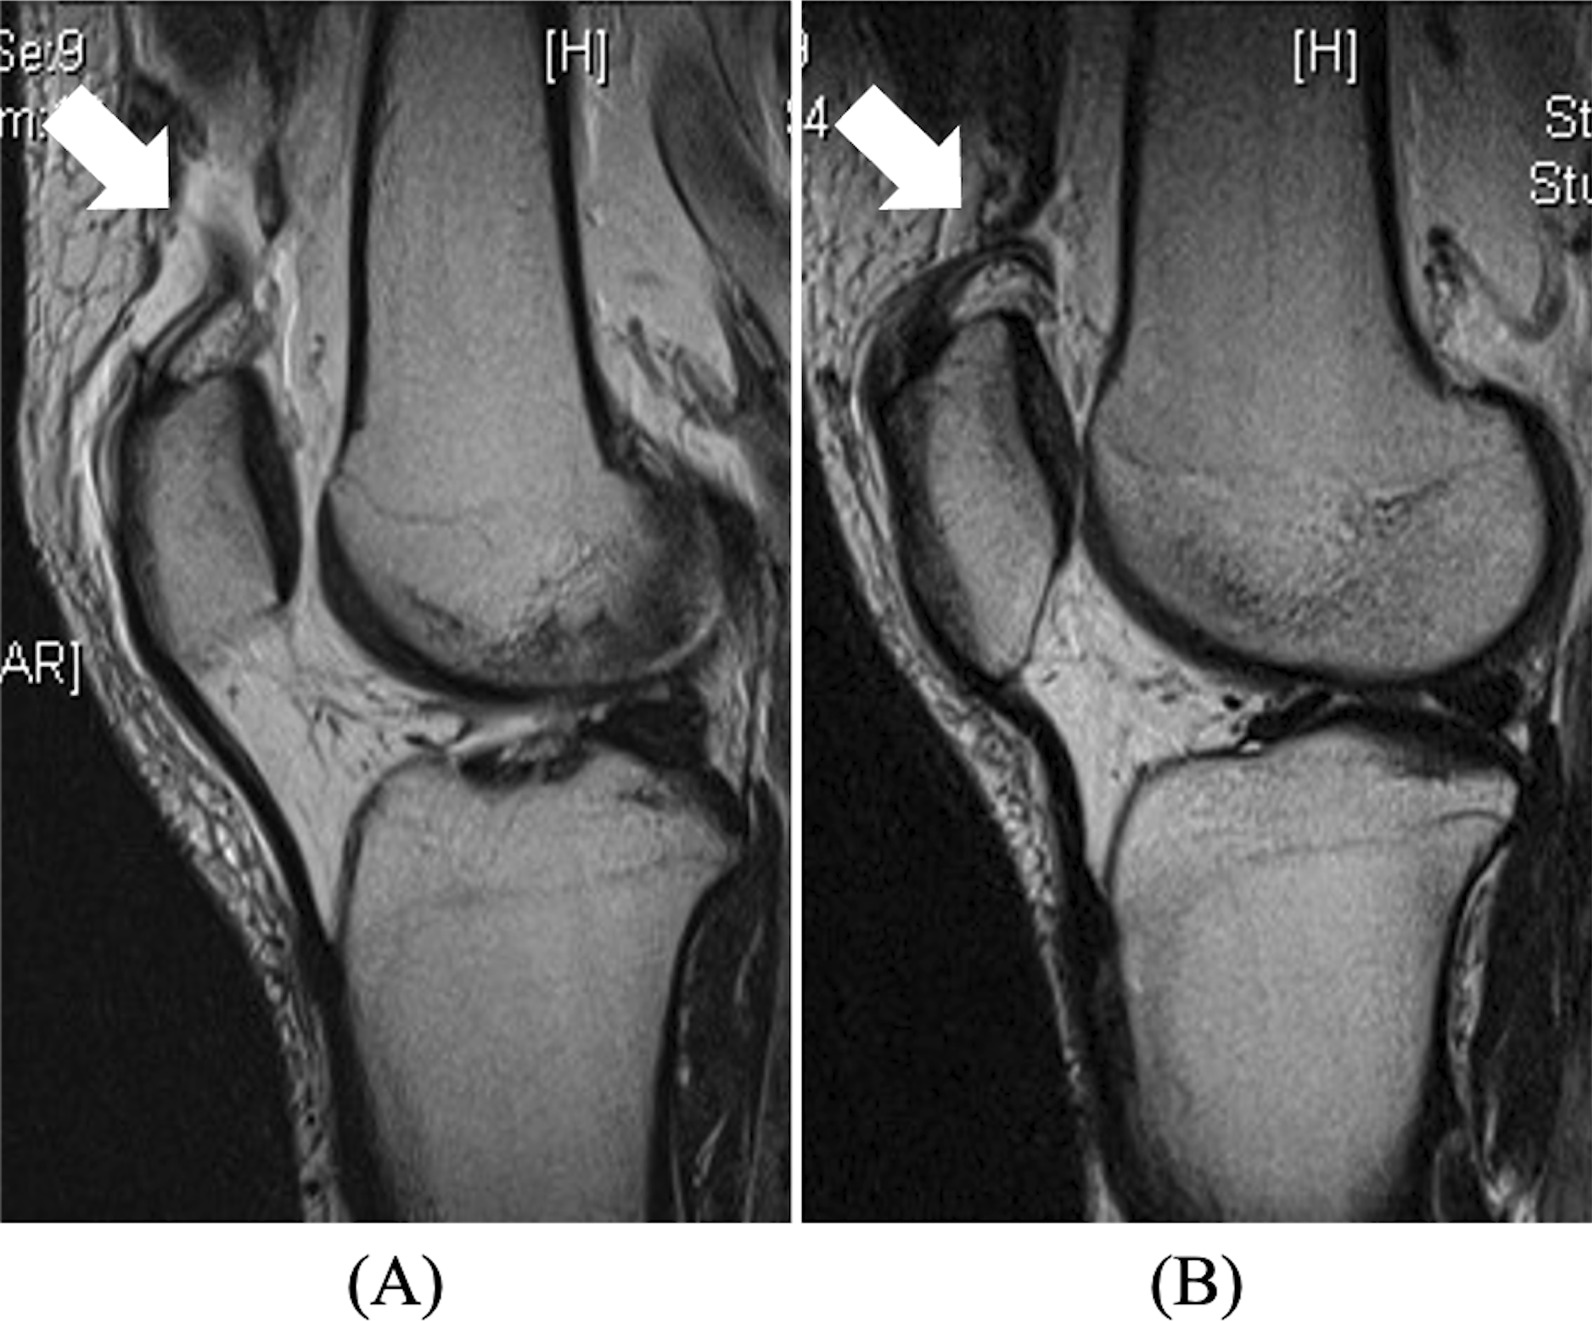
Preoperative sagittal T2-weighted imaging shows rupture of the quadriceps tendon (white arrow) of right knee (A) and left knee (B)
